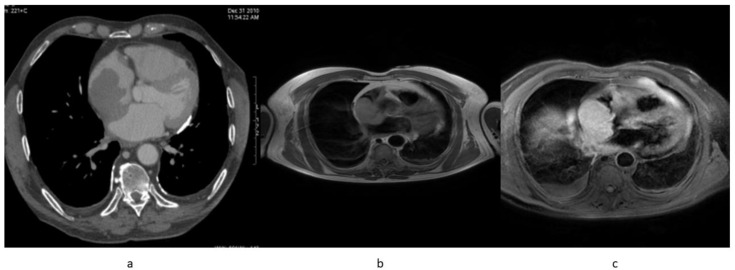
January 2020; (a) CT scan showing a right atrial mass hypointens after contast enhanced agent and apparent thickening of the left atrial wall; (b) MR with sequences BB T1, confirming the right atrial mass: (c) BBT1 delayed gadolinium images sequence showing a lesional delayed enhancement.
